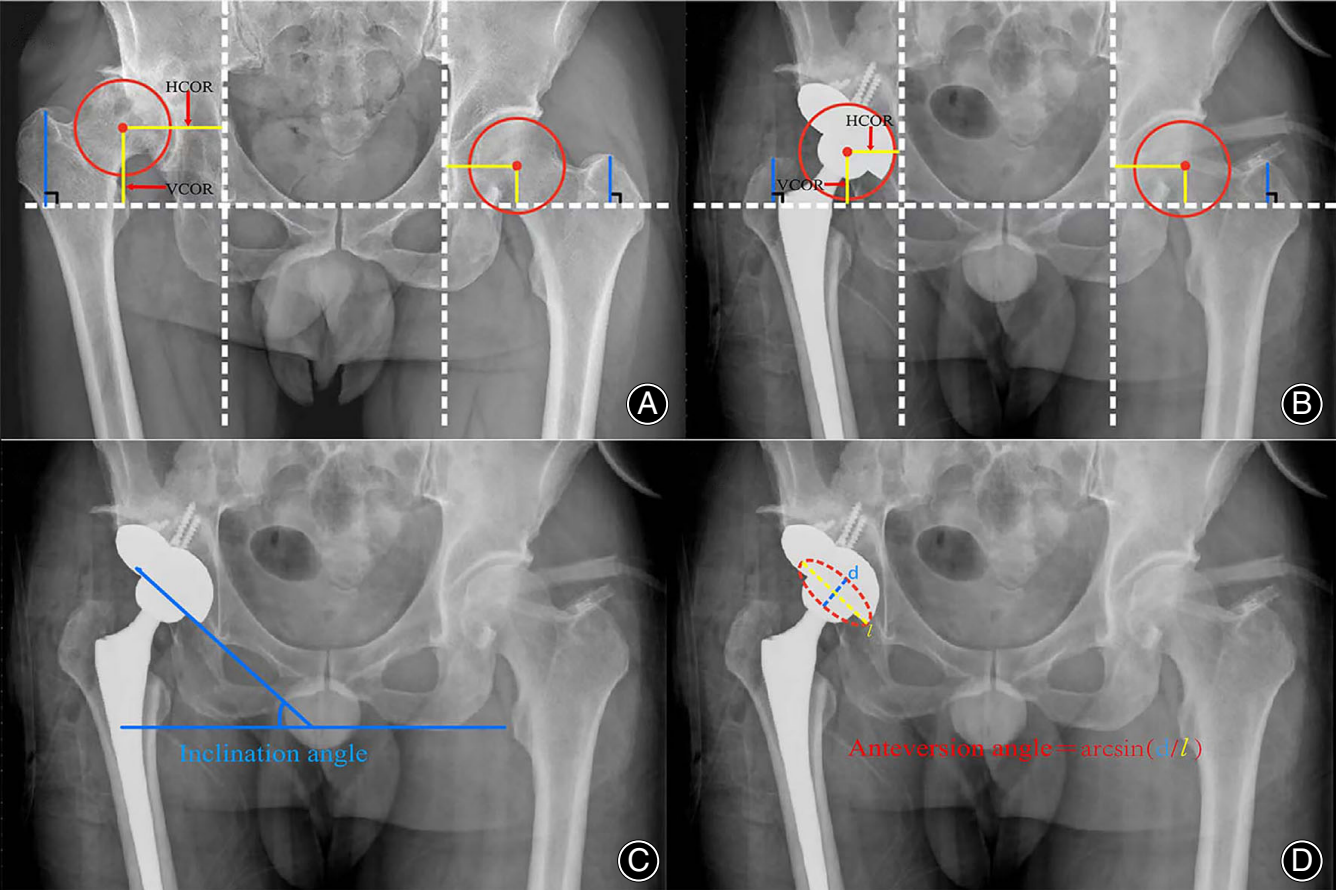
Radiographic assessments. (A) Preoperative V‐COR, H‐COR, and LLD measurements. (B) Postoperative V‐COR, H‐COR, and LLD measurements. (C) Cup inclination measurement. (D) Cup anteversion measurement.
